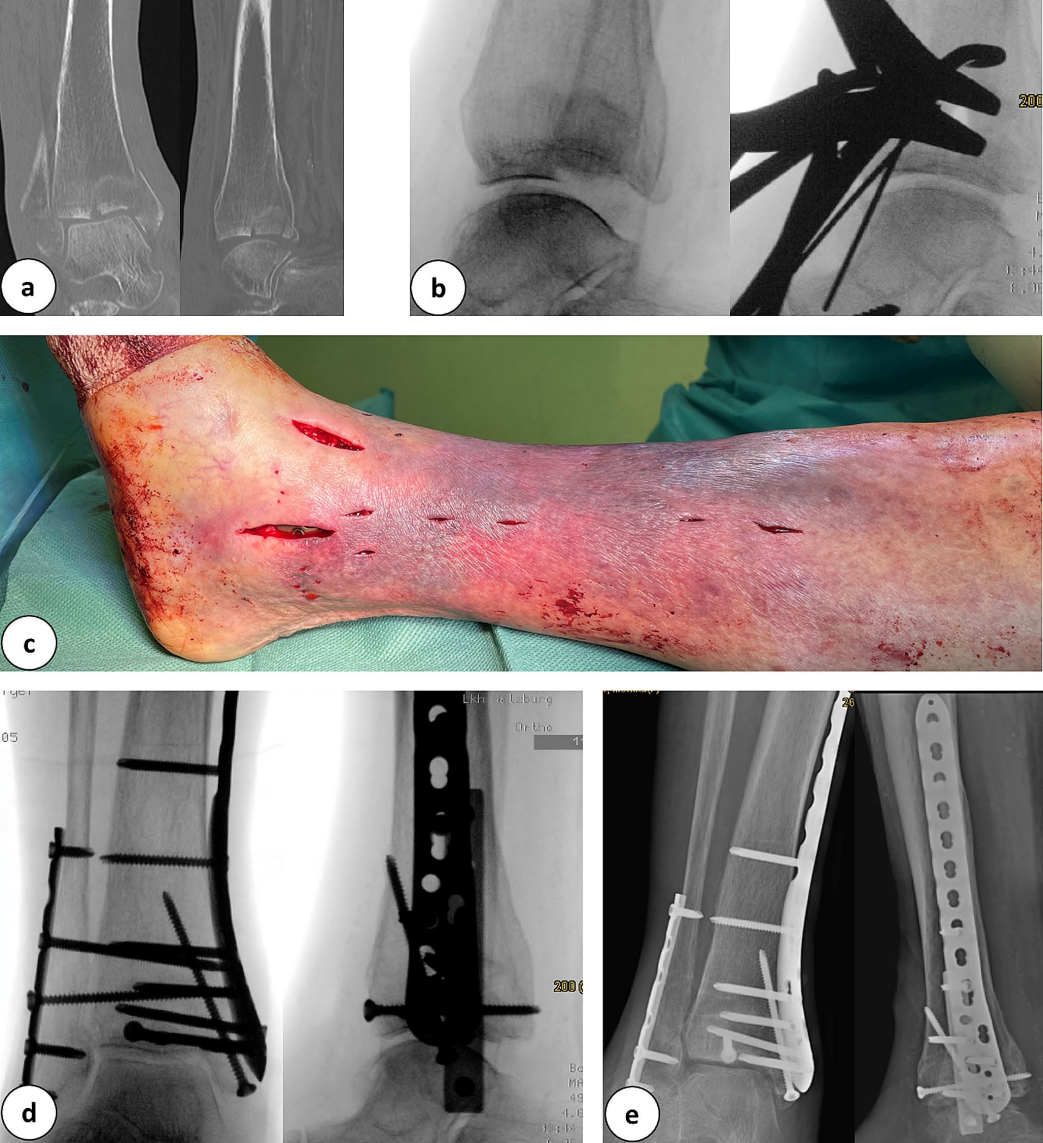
a: Anterior and lateral slices of a computed tomography scan of a multifragmentary distal tibial fracture with a pilon fracture. b: Lateral intraoperative radiographs before and after reduction of the distal tibial articular surface. c: Clinical images of the surgical approaches postoperatively before wound closure. d: Intraoperative final radiographic control after plate osteosynthesis and set screw implantation in 2 planes. e: Radiograph in 2 planes after removal of the set screw and fracture consolidation, 12 weeks postoperatively
